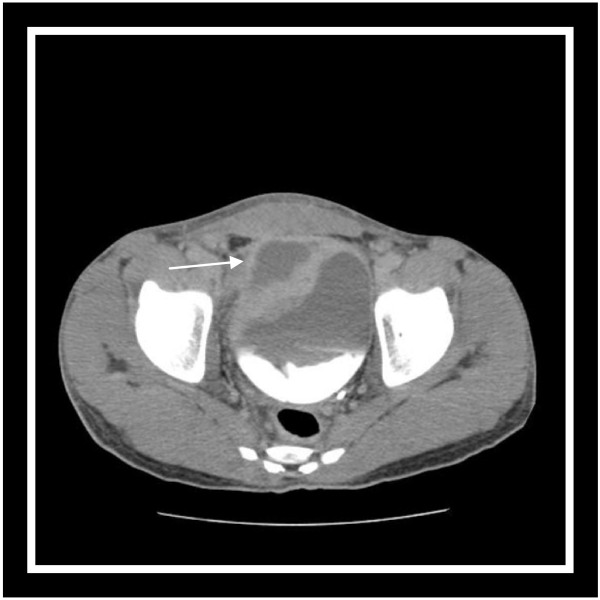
Abdominal computed tomography scan with excretory time shows hypogastric collection above the bladder, with the absence of the communication between the collection and the bladder.
